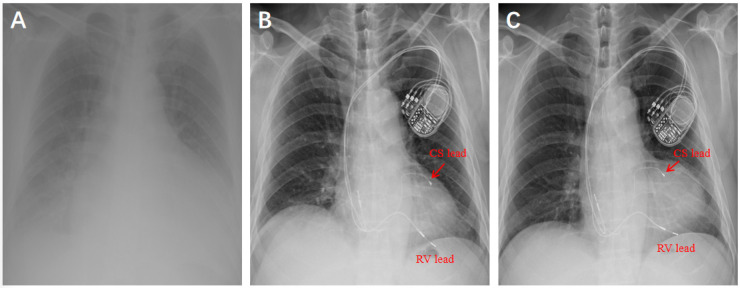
Posteroanterior chest radiograph. (A) In April 2012, Enlarged heart, pulmonary congestion. (B) In April 2013 at 1-year after CRT implatation, arrow indicates the position of the coronary sinus (CS) lead. (C) January 2025 at the 1-month follow-up of the surgery, arrow indicates the position of the coronary sinus lead.
